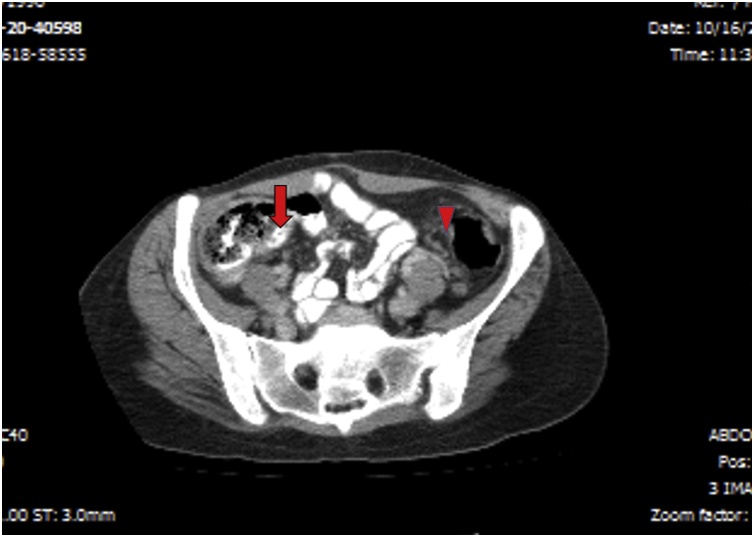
A tumor in left colon (arrow head) and ileal nodules (arrow) in abdominal CT.
